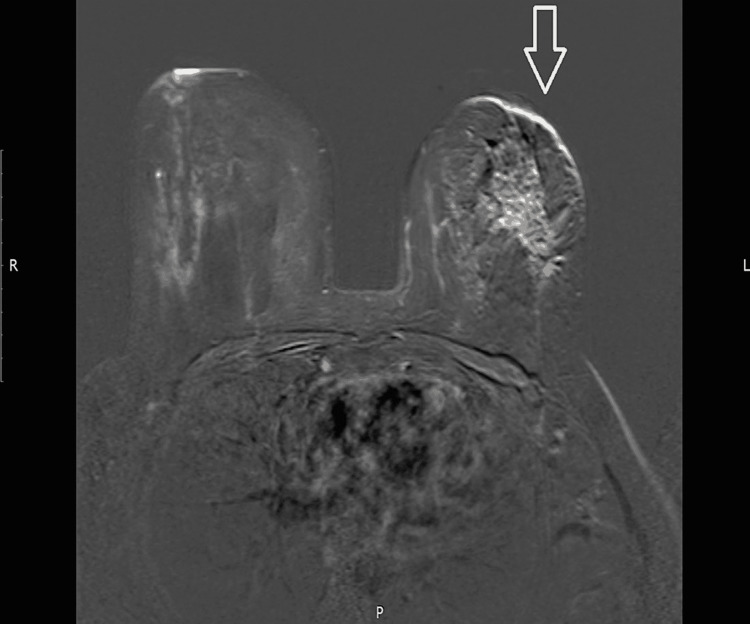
Magnetic resonance imaging shows left breast showed 4.2 x 3.3cm heterogeneously enhancing asymmetric mass like enhancement area within the left breast outer quadrant with adjacent another spiculated nodular lesion measuring 2.2cm
